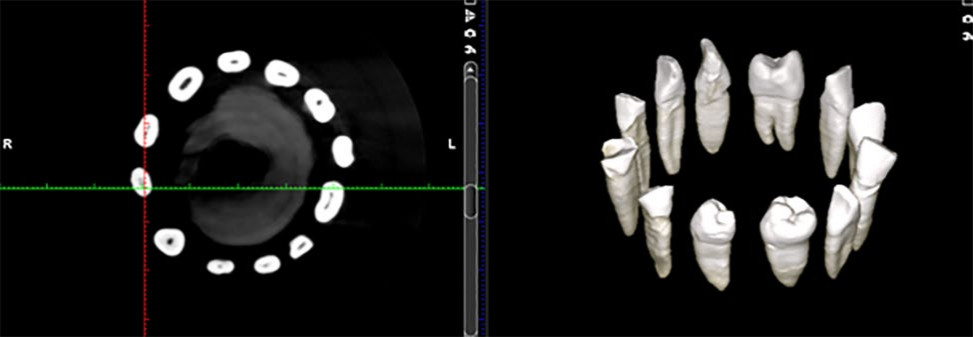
CBCT scan of the teeth inside the support structure
